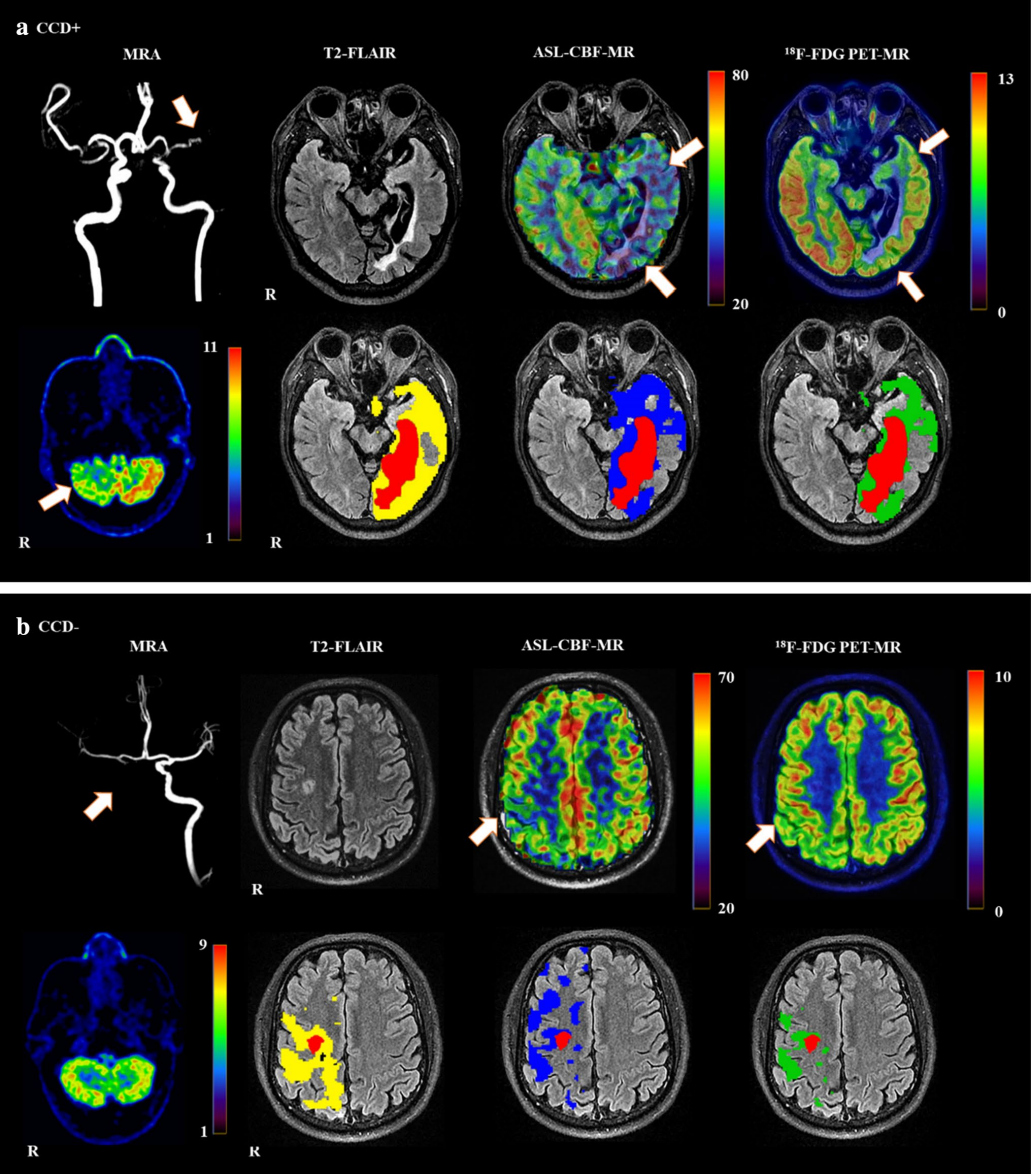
Example of a CCD-positive a and a CCD-negative b participant. a A 44-year-old man with ischemia in the left hemisphere due to left middle cerebral artery occlusion (white arrow). On arterial spin labeling (ASL)—cerebral blood flow (CBF) imaging and ^18^F-FDG positron emission tomography (PET), supratentorial hemodynamic and metabolic impairment may be seen in the left hemisphere (white arrows). Crossed cerebellar diaschisis (CCD) on ^18^F-FDG PET images shown of the cerebellum (white arrow). b A 62-year-old man with ischemia in the right paracentral hemisphere due to right internal carotid artery occlusion (white arrow). On ASL-CBF imaging and ^18^F-FDG PET, supratentorial metabolic and hemodynamic impairment is shown in the right hemisphere (white arrows), No CCD was observed on the cerebellar ^18^F-FDG PET image. Red—infarct zone in the supratentorial hemisphere. Yellow—decreased blood flow regions. Blue—decreased metabolism regions. Green—co-decreased regions
